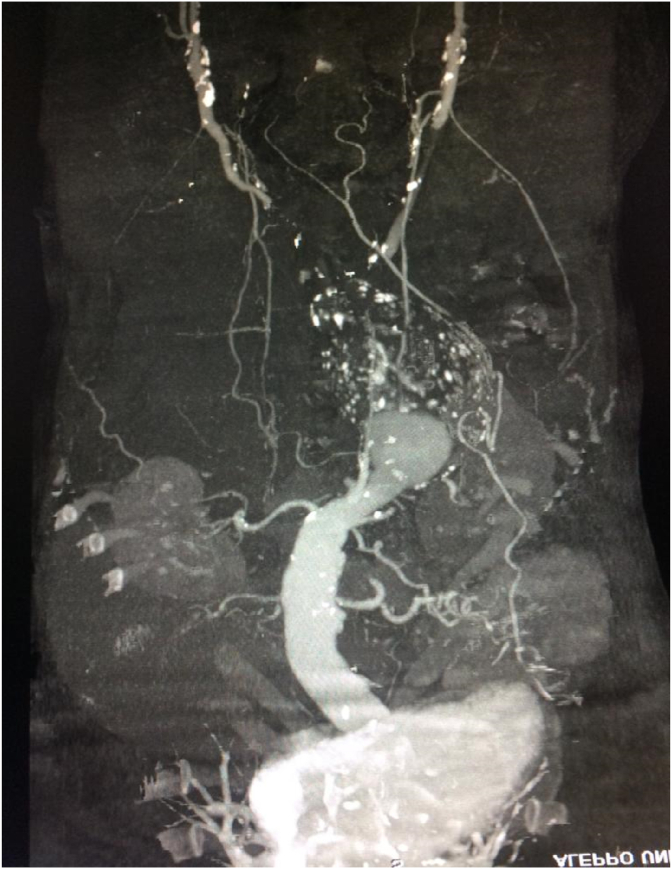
Multislice CT scan showing the dimensions of the aneurysm.
